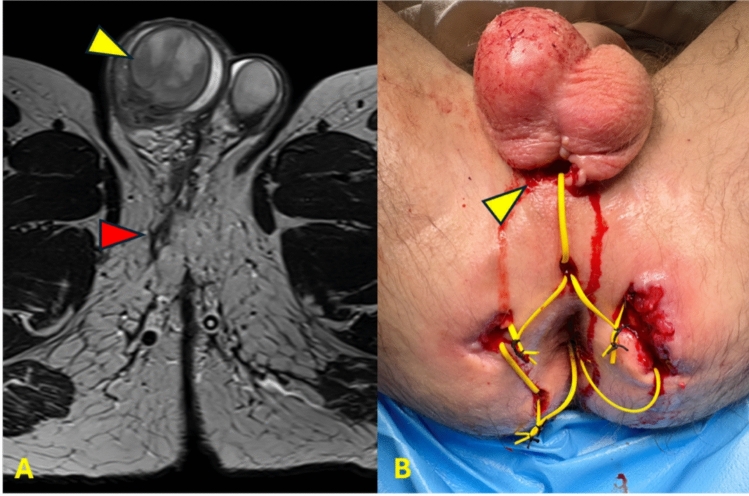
MRI revealed scrotal and testicular abscesses (yellow arrow) and fistulous tracts reaching to the base of the scrotum (red arrow) (A). Surgical rationalization with placement of a total of six setons for drainage of the fistulous network, one reaching the base of the scrotum (yellow arrow) (B). © Cleveland Clinic Foundation, 2026
